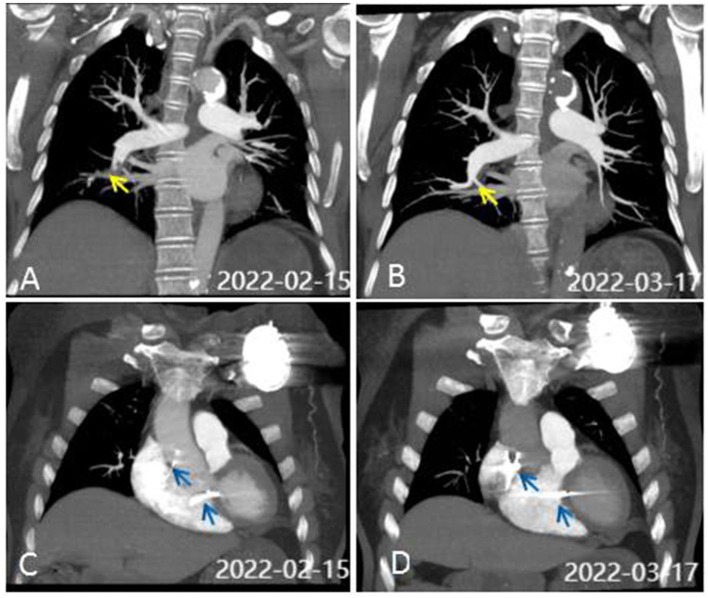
CT scan of the pulmonary artery. (A) CTA on admission showed pulmonary embolism (PE) in the right lower pulmonary arterial branch. Perfusion defects are identified by yellow arrows. (B) CTA was normal after 1 month of anticoagulant therapy. (C, D) show the positions of the RV and atrial leads (blue arrows) on PA view on admission (C) and 1 month later (D).
